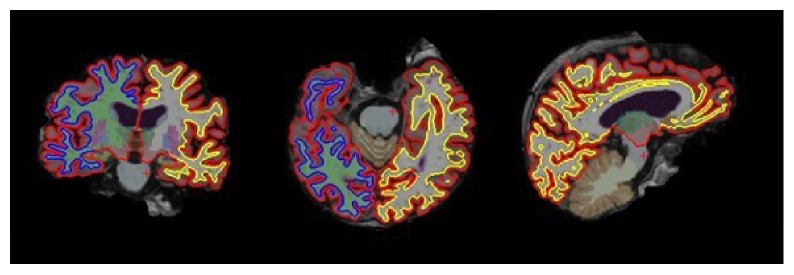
Segmentation of brain MR images for volumetric study.
